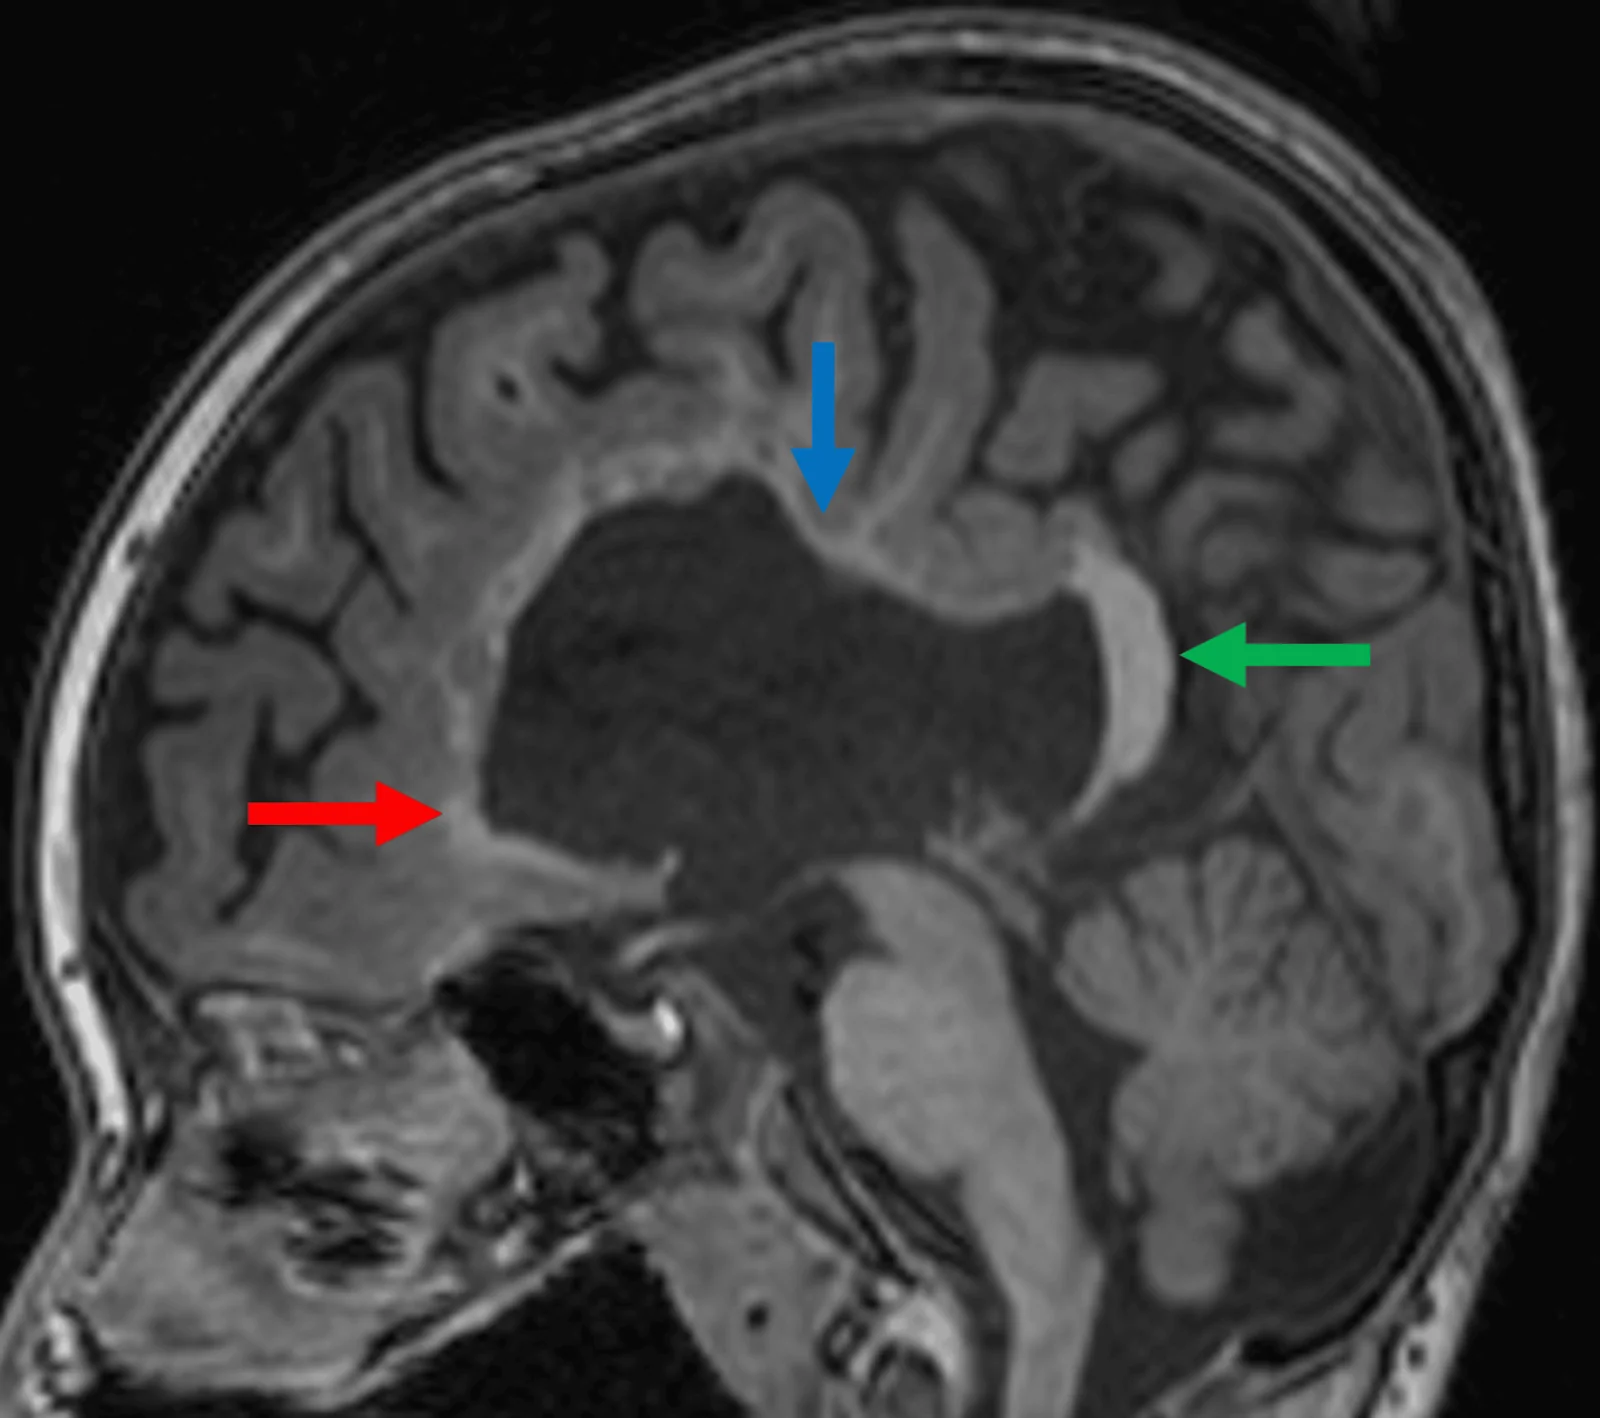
MRI image demonstrating absence of the body of the corpus callosum and hypoplasia of the genu and splenium of the corpus callosum Sagittal T1-weighted MRI image without intravenous contrast shows hypoplastic genu of the corpus callosum (red arrow), absent body of the corpus callosum (blue arrow), and hypoplastic splenium of the corpus callosum (green arrow).
